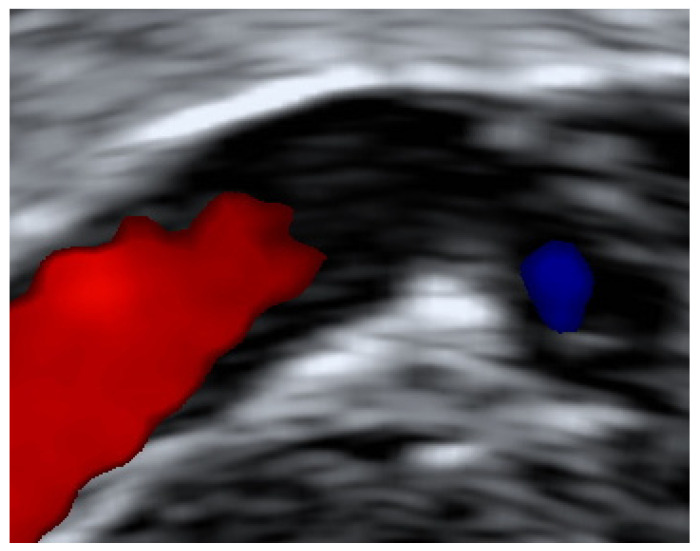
Colour Doppler transvaginal image of fibrosis within uterine vein.
